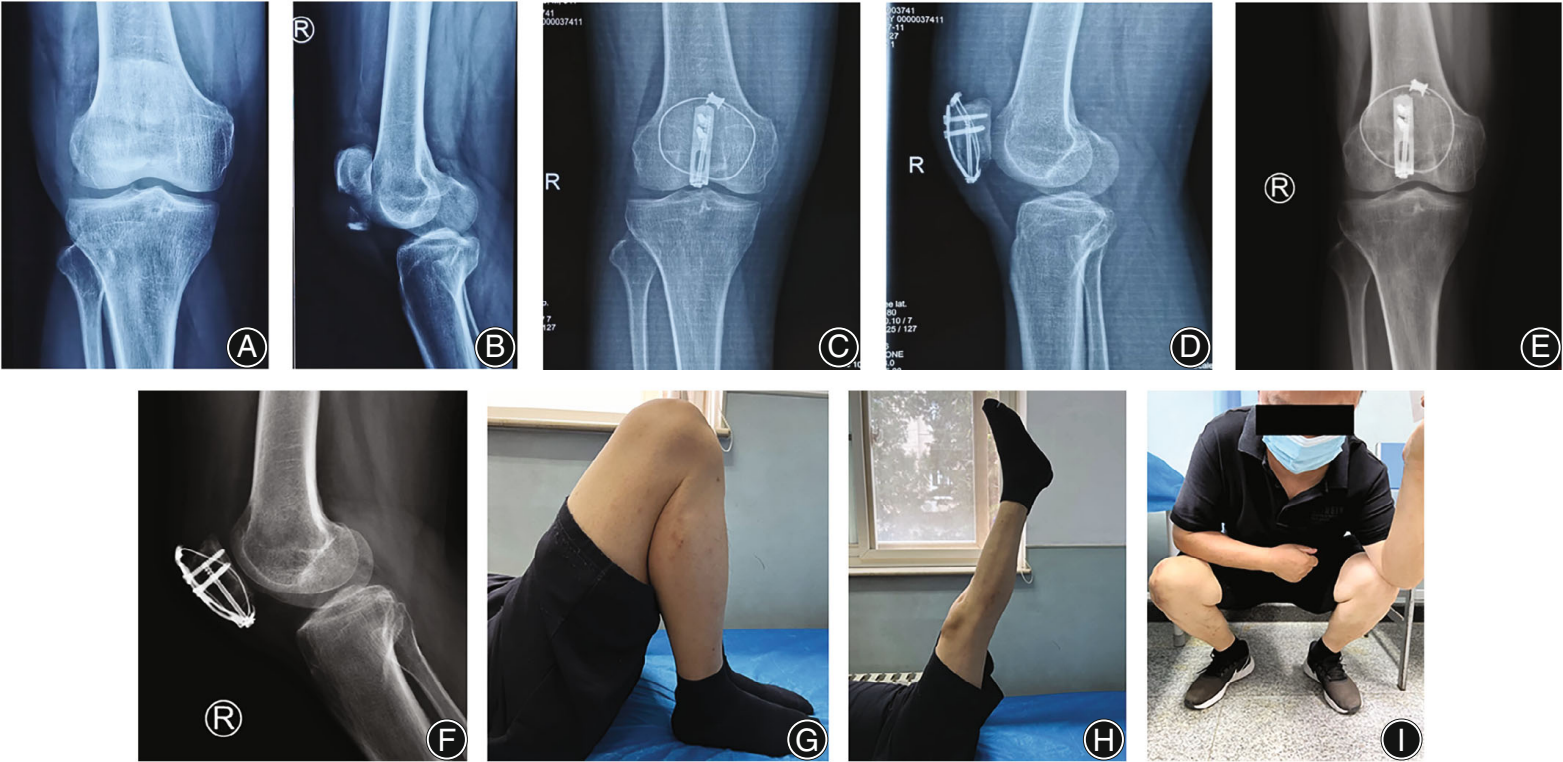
Case example (case 4, 55 years, male, right side). Preoperative anteroposterior (A) and lateral (B) radiographs showed a displaced inferior patellar pole fracture. Postoperative anteroposterior (C) and lateral (D) radiographs. Radiographs (E‐F) and function (G–I) at 2 years follow‐up
